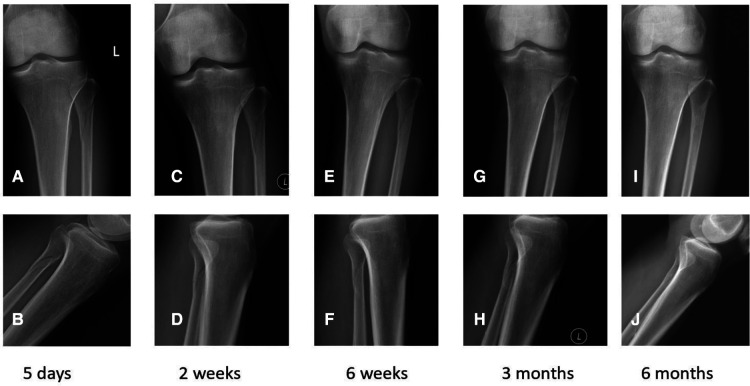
Frontal (A, C, E, G, I) and lateral (B, D, F, H, J) view CT images of the proximal tibia (5 days, 2 weeks, 6 weeks, 3 months, and 6 months after the injury).
